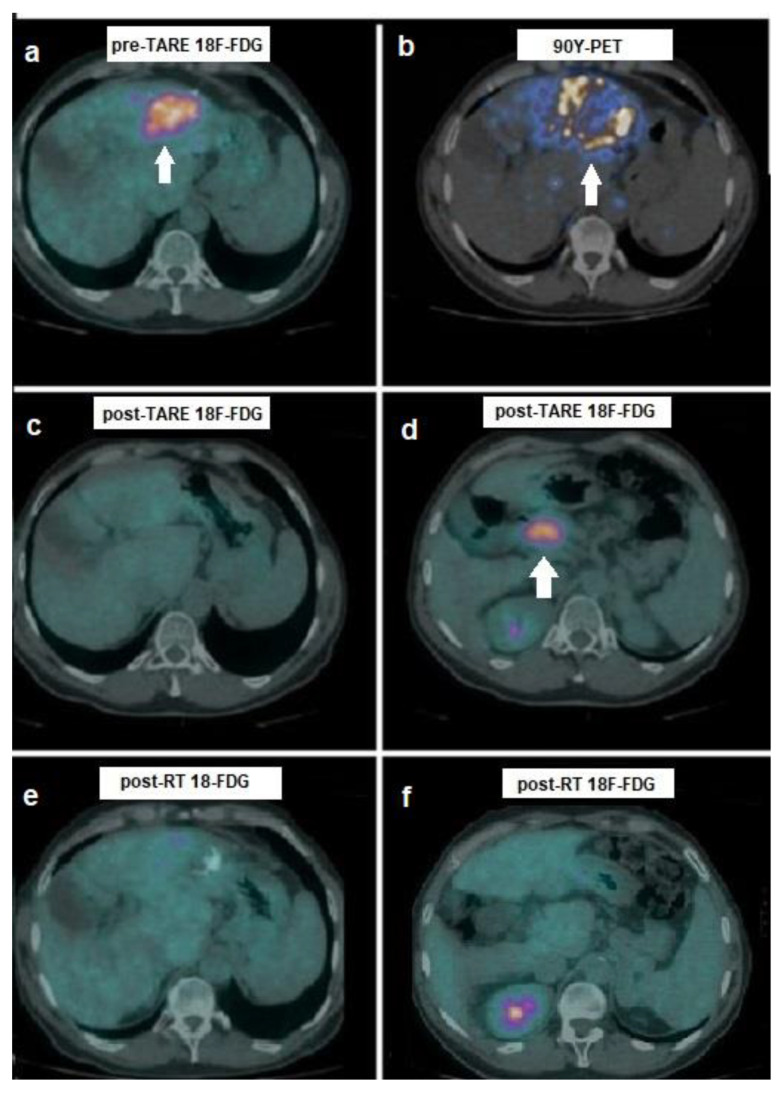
A 51-year-old affected by HCV-related cirrhosis and HCC in the left hepatic lobe, with portal vein invasion, progressive during systemic therapy with sorafenib. On pre-treatment examination, HCC resulted ^18^F-FCH-negative, but (a) intensely positive on ^18^F-FDG PET/CT (arrow, SUVmax 17.7). (b) After selective catheterization of HCC’s arterial feeder, he was administered with ^90^Y-microspheres: ^90^Y-PET showed a peripheral pattern of accumulation with respect to HCC lesion (arrow). At 8-weeks post-treatment ^18^F-FDG PET/CT, a complete regression of HCC in the left lobe was evident (c), although the onset of an intensely hypermetabolic celiac lymph adenopathy was detected ((d), arrow). Therapeutic decision was stereotactic RT on the ^18^F-FDG-positive metastasis. On follow-up ^18^F-FDG PET/CT carried out at 2 months after RT, complete metabolic response was registered both in the hepatic lesion (e) and in nodal metastasis (f). Overall survival resulted in 36 months.
